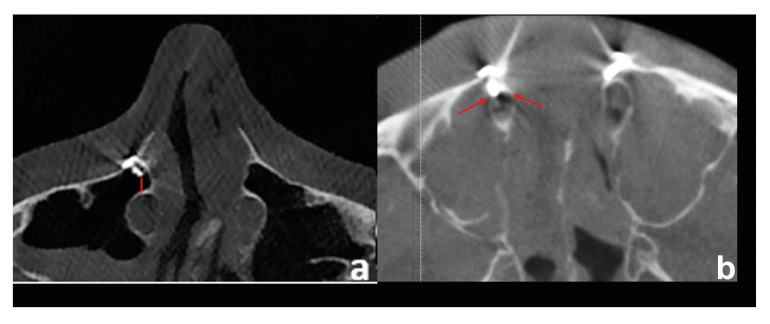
Postoperative CBCT images (a: The distance between the canal and the screws, b: The perforation of the bony nasolacrimal canal).
